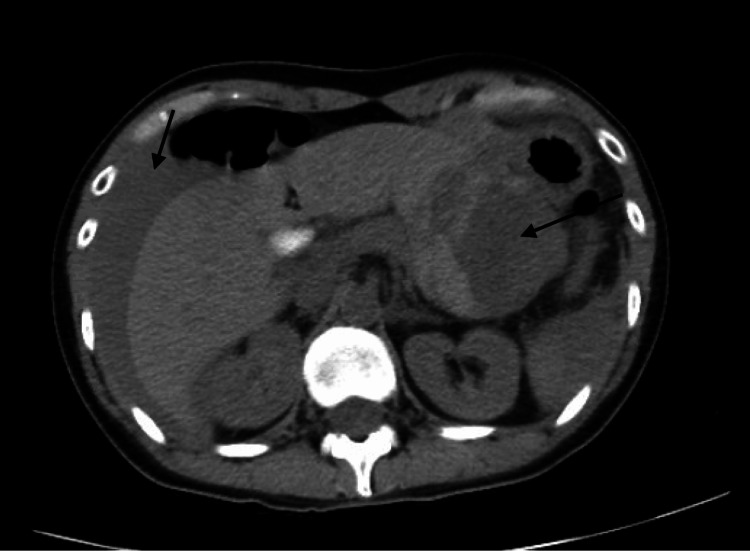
CT angiography performed 14 hours after the initial presentation showing a mass intimately associated with the stomach with associated hypervascularity consistent with a GIST. There is an increased amount of fluid within the abdomen concerning for hemoperitoneum. GIST = gastrointestinal stromal tumor
